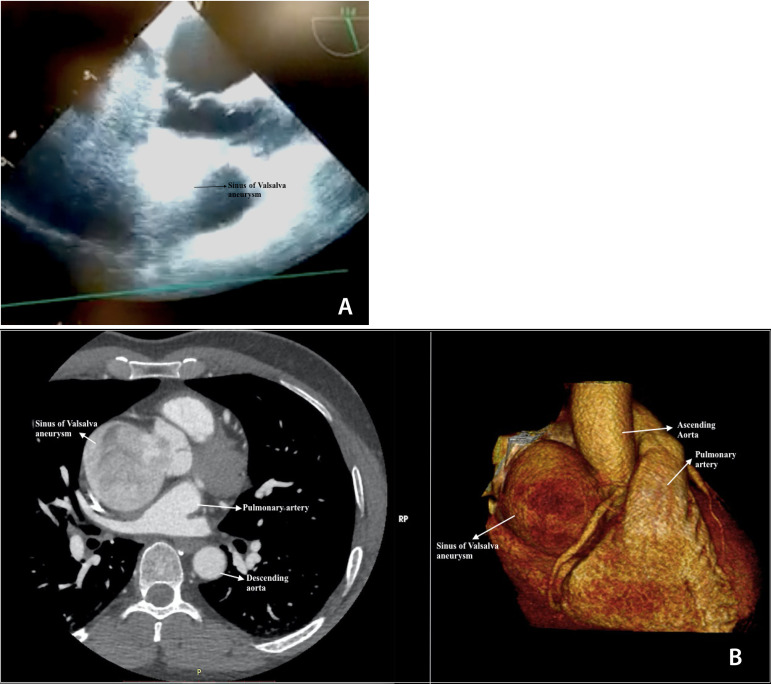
A) Echocardiography indicates dilatation of the non-coronary sinus; B) Computer tomography shows the aneurysm of the non-coronary sinus of Valsalva.
